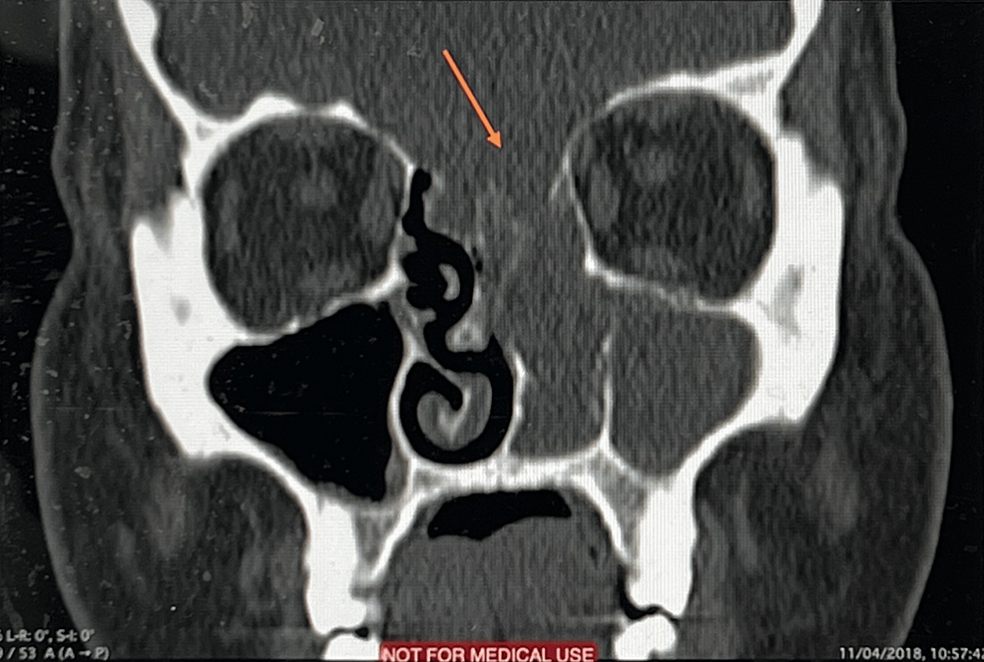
Coronal computed tomography showing skull base defect.
